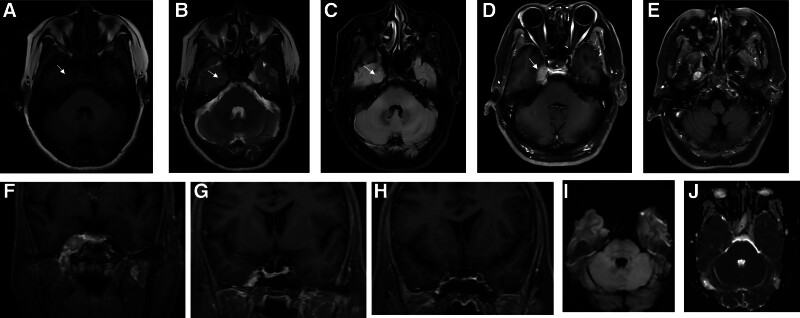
(A) T1WI, (B) T2WI, and (C) T2-FLAIR sequences demonstrate the lesion (arrow) exhibiting isointensity to brain parenchyma. (D and F) Axial and coronal T1-weighted contrast-enhanced MRI show that the parasellar mass-like lesion had obvious heterogeneous enhancement, with unclear boundary from the trigeminal nerve, and invaded the cerebellopontine angle area through the “Meckel cave.” (E) Axial T1-weighted enhanced MRI shows tumor extension through the foramen ovale into the infratemporal fossa. (G and H) Coronal T1-weighted enhanced MRI confirms involvement of the lateral wall of the right cavernous sinus. (I and J) DWI and ADC demonstrate mild diffusion restriction, evidenced by partially hyperintense DWI signals and correspondingly hypointense ADC values within the lesion. ADC = apparent diffusion coefficient, DWI = diffusion-weighted imaging, MRI = magnetic resonance imaging.
